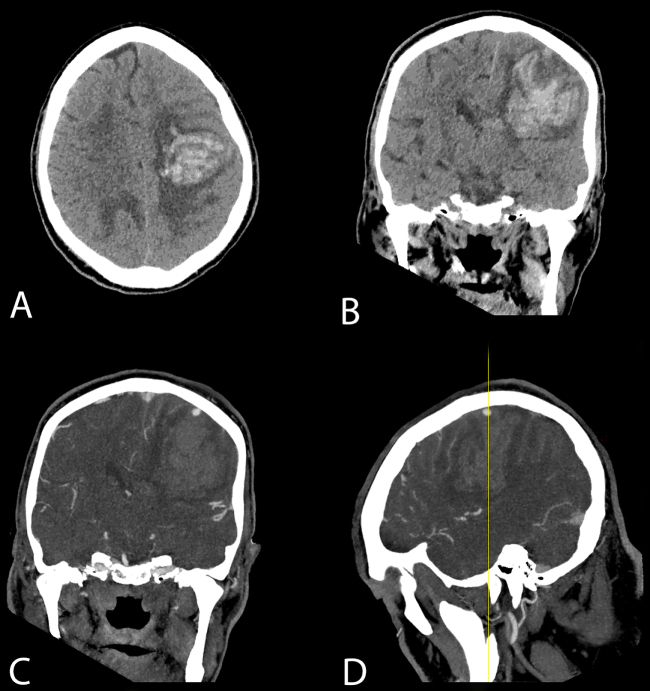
Pre-operative non-contrast CT brain – axial (A), coronal (B) views, and pre-operative CT brain angiogram – coronal (C), sagittal (D) views.
